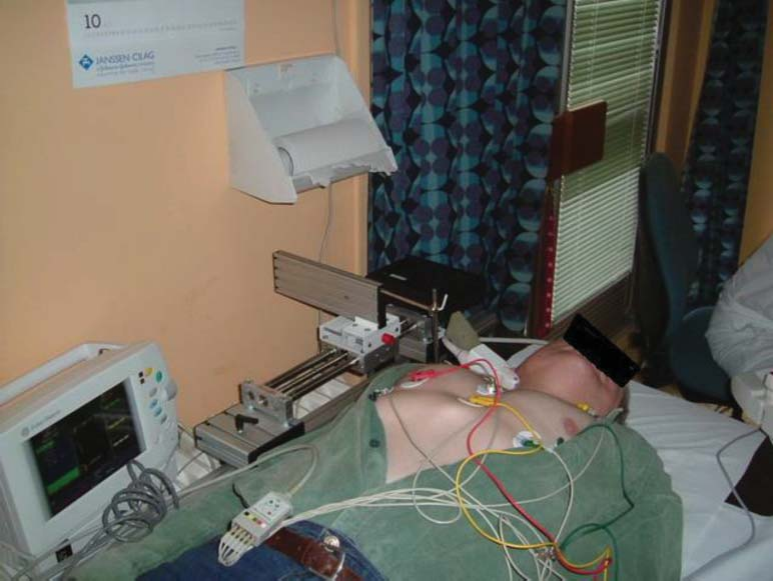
Configuration of the proposed device for the 3D ultrasound display and measurement of the atherosclerotic plaque.
